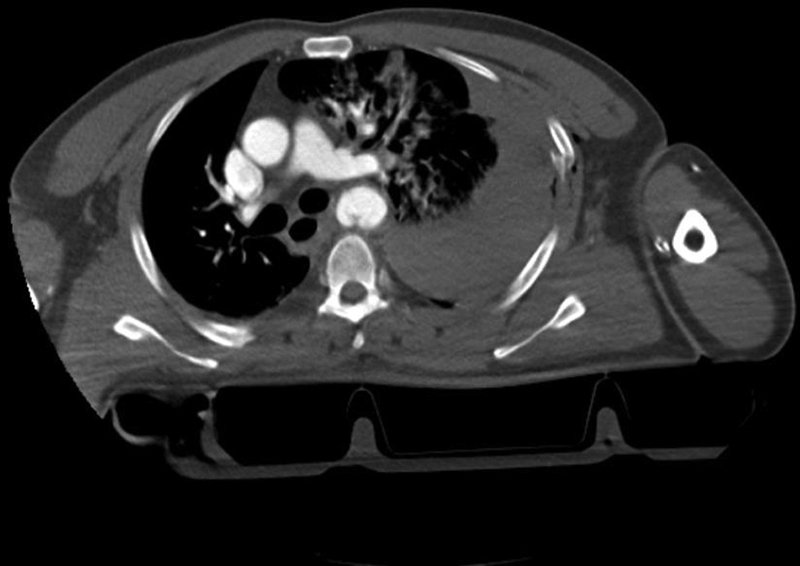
Computed tomography angiogram demonstrating aortic isthmus pseudoaneurysm, mediastinal hematoma and left hemothorax after blunt trauma.
